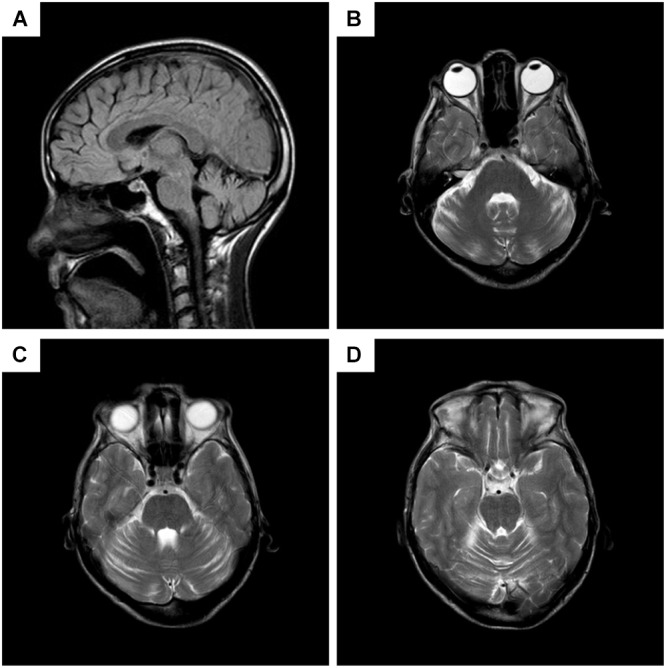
Brain MRI of the patient. (A) Sagittal T2 fluid attenuated inversion recovery (FLAIR) imaging, showing decreased volume of the anterior lobe of cerebellum, while cerebral lobes, periventricular white matters, and the brain stem are not atrophied. (B–D) Different planes of axial T2 imaging, presenting slim cerebellar gyrus and deepened cerebellar sulcus in anterior lobe.
